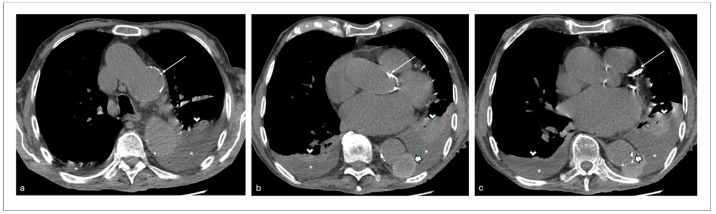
An 85-year-old man with severe renal insufficiency (serum creatinine level 266 µmol/L, normal range 59–104 µmol/L, and glomerular filtration rate 18 mL/min/1.73 m^2^) and without any history of recent trauma underwent a plain chest CT scan (CT; CT scanner General Electrics, GE, Revolution EVO 64, GE Healthcare, Chicago, IL, USA) for persistent cough and shortness of breath for months. The axial unenhanced chest CT revealed bilateral pleural effusion (white asterisks in (a–c)) and atelectasis (white arrowhead in (a–c)). Moreover, a large aneurysm of the aortic arch and diffuse vascular calcifications (thin white arrow in (a–c)) were evident. As an incidental finding, a round inhomogeneous lesion was detected near to the descending thoracic aorta (white arrow in (b)), with outpouching calcifications (white arrow in (c))). Given the well-defined, rounded shape, its location near the descending thoracic aorta, the displaced calcification, and the hyperdense signal, it was considered as a possible pseudoaneurysm. Although the hyperdense peripheral component, compatible with a hematoma, the smooth margins, the proximity to the aorta, and the outpouching calcifications were suggestive of a pseudoaneurysm, further assessment was needed. It should also be highlighted that the patient was recently checked with an echocardiography which assessed the presence of the aneurysm in the ascending aorta but did not mention the pseudoaneurysm. Since the patient could not undergo a contrast-enhanced examination to better characterize these findings, a thoracic MR was required.
